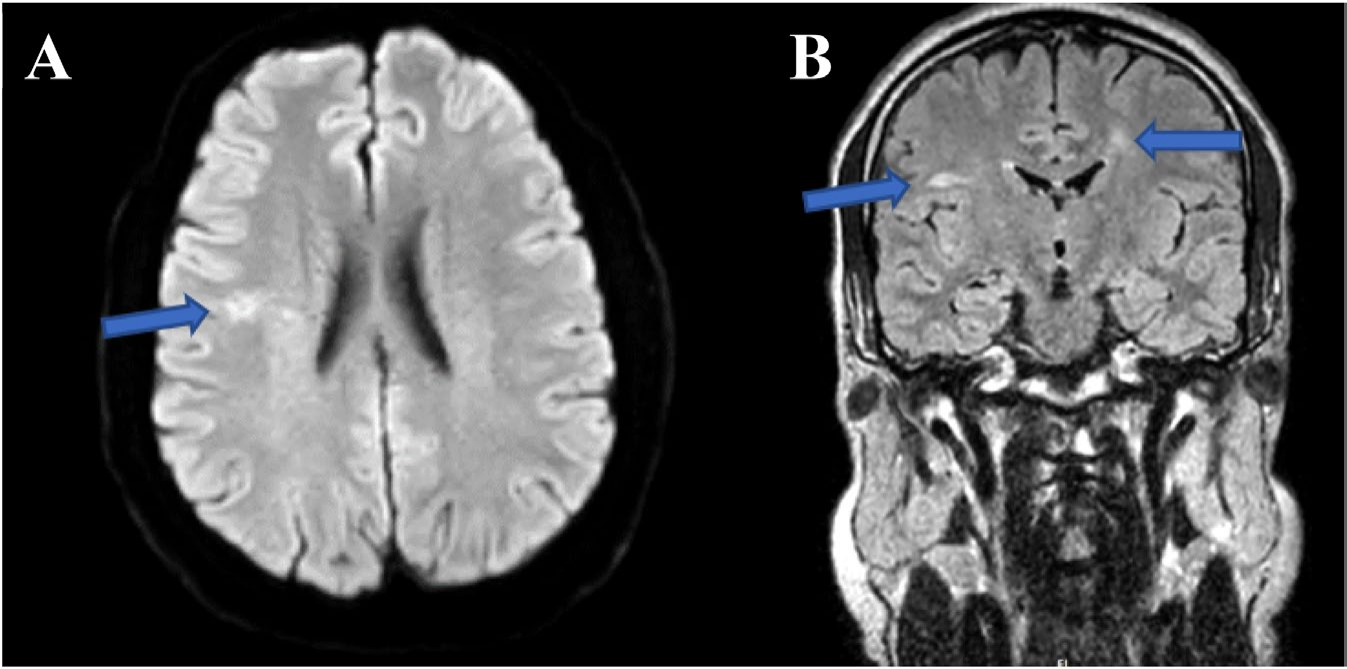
MRI T2 weighted axial (A) and coronal (B) images revealed hyperintense white matter lesions (Blue arrows).
